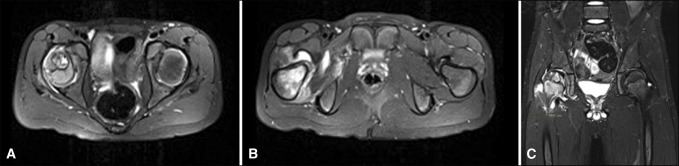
A, Radiograph showing axial T2 (repetition time 3230ms, echo time 4800ms) MRI of the pelvis revealing a well-defined lesion in the femoral epiphysis with a cystic component that measures 19.3 × 17.7 mm. B, Radiograph shows hyperintense marrow changes diffusely within the femoral head with associated joint effusion and soft-tissue edema. C, Radiograph showing coronal inversion recovery MRI (repetition time 4650ms, echo time 4600ms) demonstrating a well-defined lesion with1 hypointense lesion in the epiphysis with a sclerotic border and extensive marrow bright signal within the metaphysis and joint effusion. MRI = magnetic resonance imaging.
